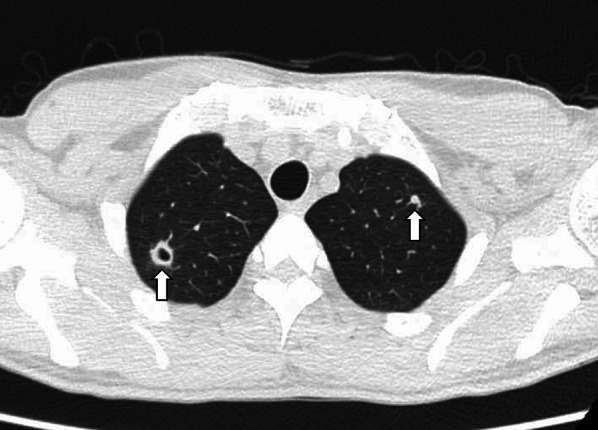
Image of lung computed tomography (CT). Cavitary lesions noted in the apices of both lungs (arrows)
